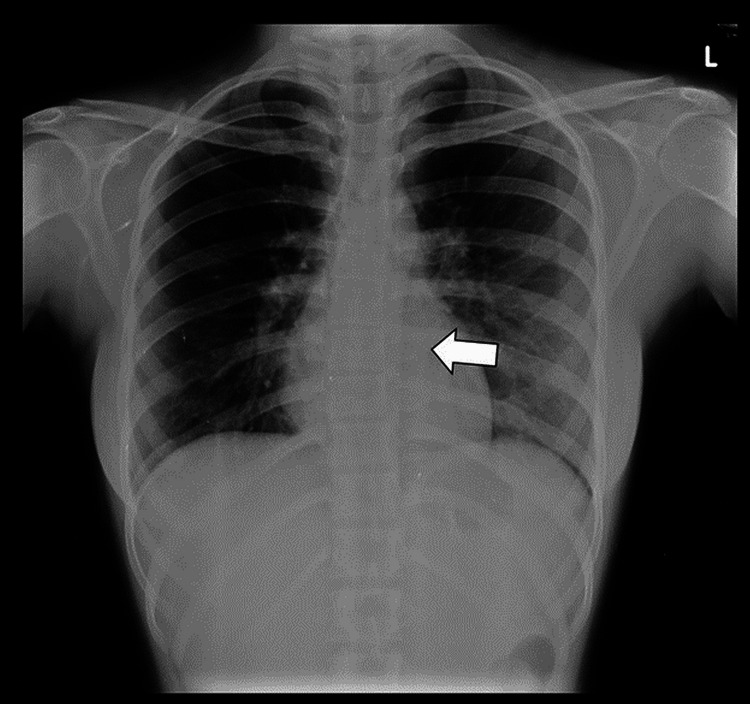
Chest X-ray (PA view) The X-ray shows a mild widening of the left paravertebral stripe in the lower thoracic region (arrow) signifying soft tissue involvement. No significant lung parenchymal or rib involvement was evident. PA: Posteroanterior
